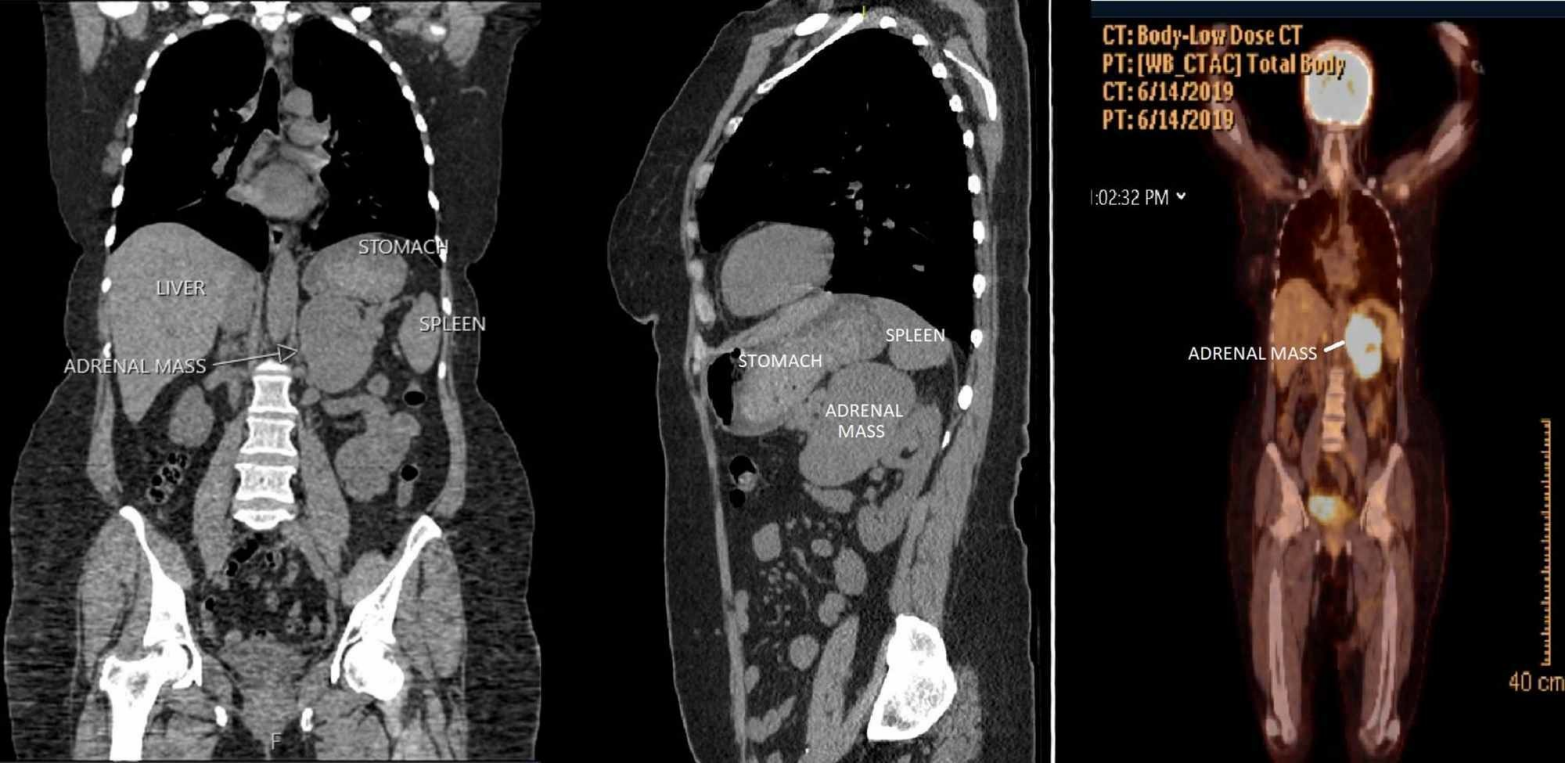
Abdominal computed tomography (CT) view with coronal (A) and sagittal section (B); positron emission tomography-CT (PET-CT) view with coronal section (C)
